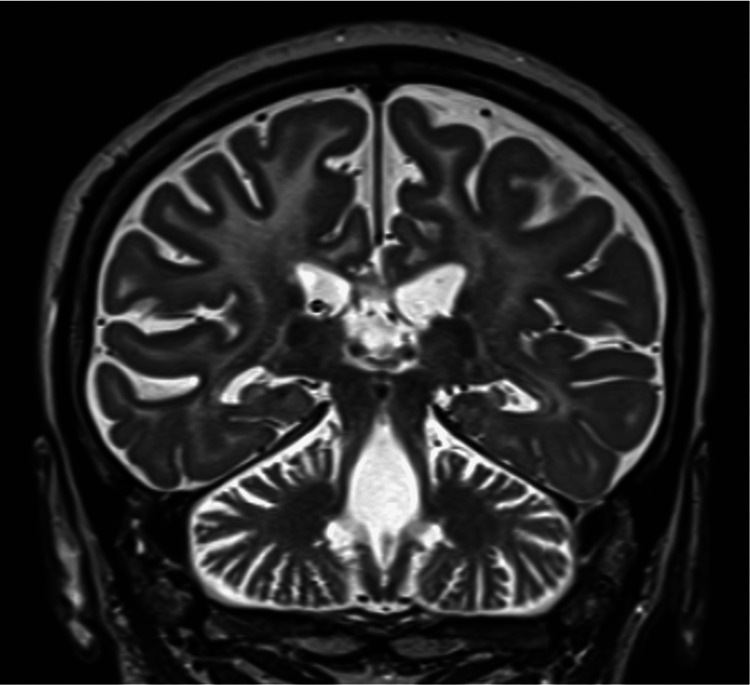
Coronal T2 Weighted Imaging Coronal T2-weighted image obtained at the level of posterior bodies of lateral ventricles demonstrate diffuse marked cerebral and cerebellar volume loss, with persistent extensive, confluent T2 hyperintense signal within the white matter.
